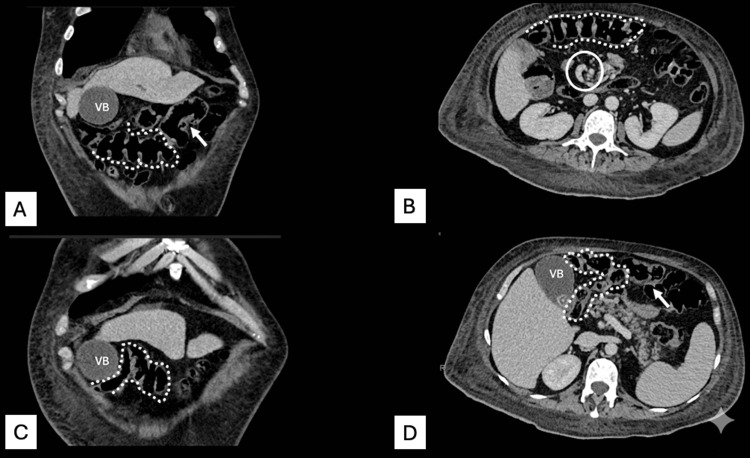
Ascending colon Contrast-enhanced CT in the venous phase demonstrating the ascending colon (dashed line) located in the epigastrium and right hypochondrium. It courses from left to right (A, B) and subsequently from anterior to posterior, medial to the gallbladder (VB) (C, D) (circle in B: mesenteric vessels; thin arrow in A and D: cecum; VB in A, C, and D: gallbladder).
